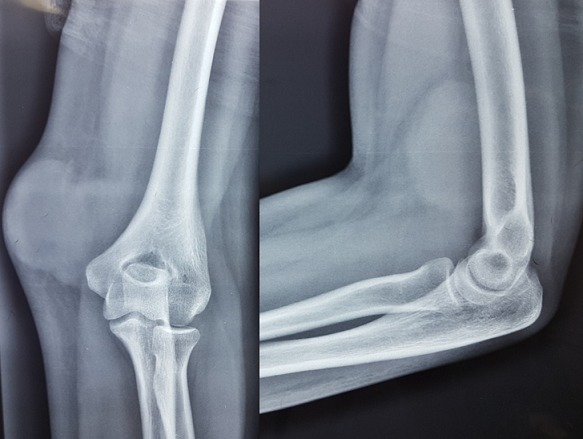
Radiographie du coude gauche face et profil: absence de lésion osseuse sous-jacente ou corps étranger
